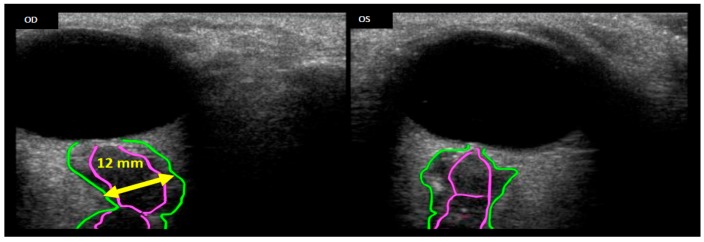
Remotely guided on-orbit ultrasound images showing the OD (right eye) Optic Nerve Sheath Diameter (ONSD, green outline) distended to approximately 12 mm, thus suggesting increased Intracranial Pressure (*ICP*). Comparing the optic nerve (pink outline) of the right eye with the left (OS) eye, a kink can clearly be seen in the OD optic nerve. Source: NASA Longitudinal Study of Astronaut Health.
